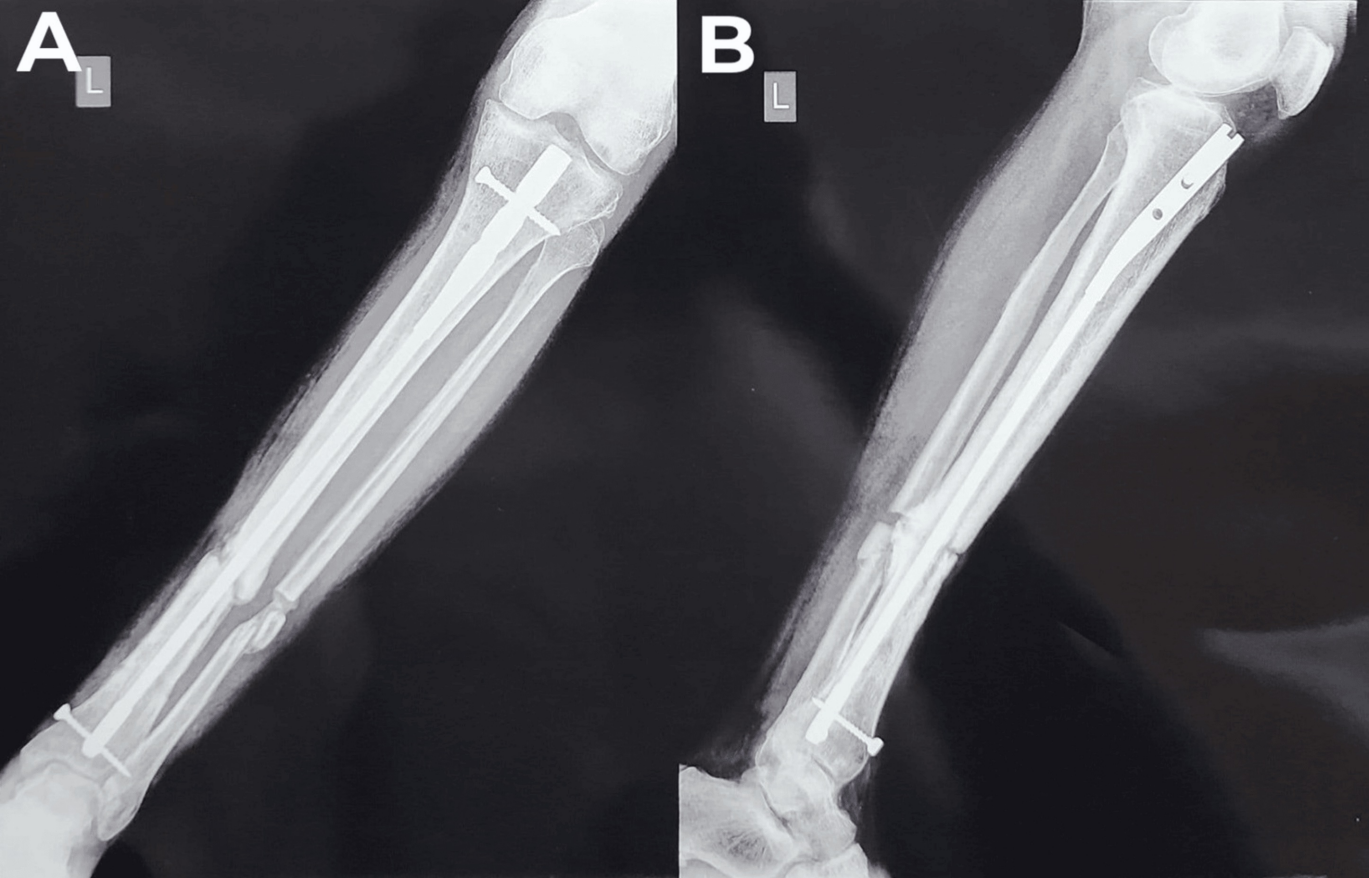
A) A left tibia and fibula AP X-ray with increased callus deposit at the non-union site. B) A left tibia and fibula lateral X-ray with increased callus deposit at the non-union site. AP: anterior posterior
